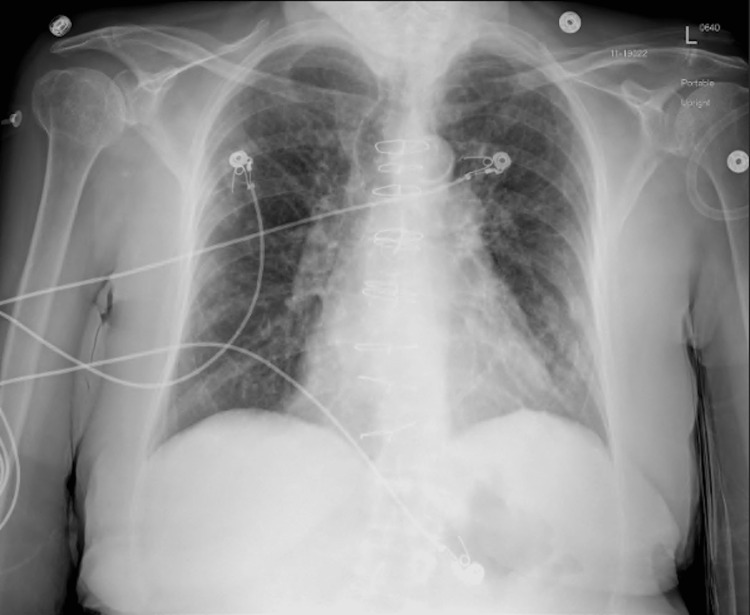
Chest X-ray on second admission was significant for worsening of interstitial infiltrates with upper lobe prominence.
